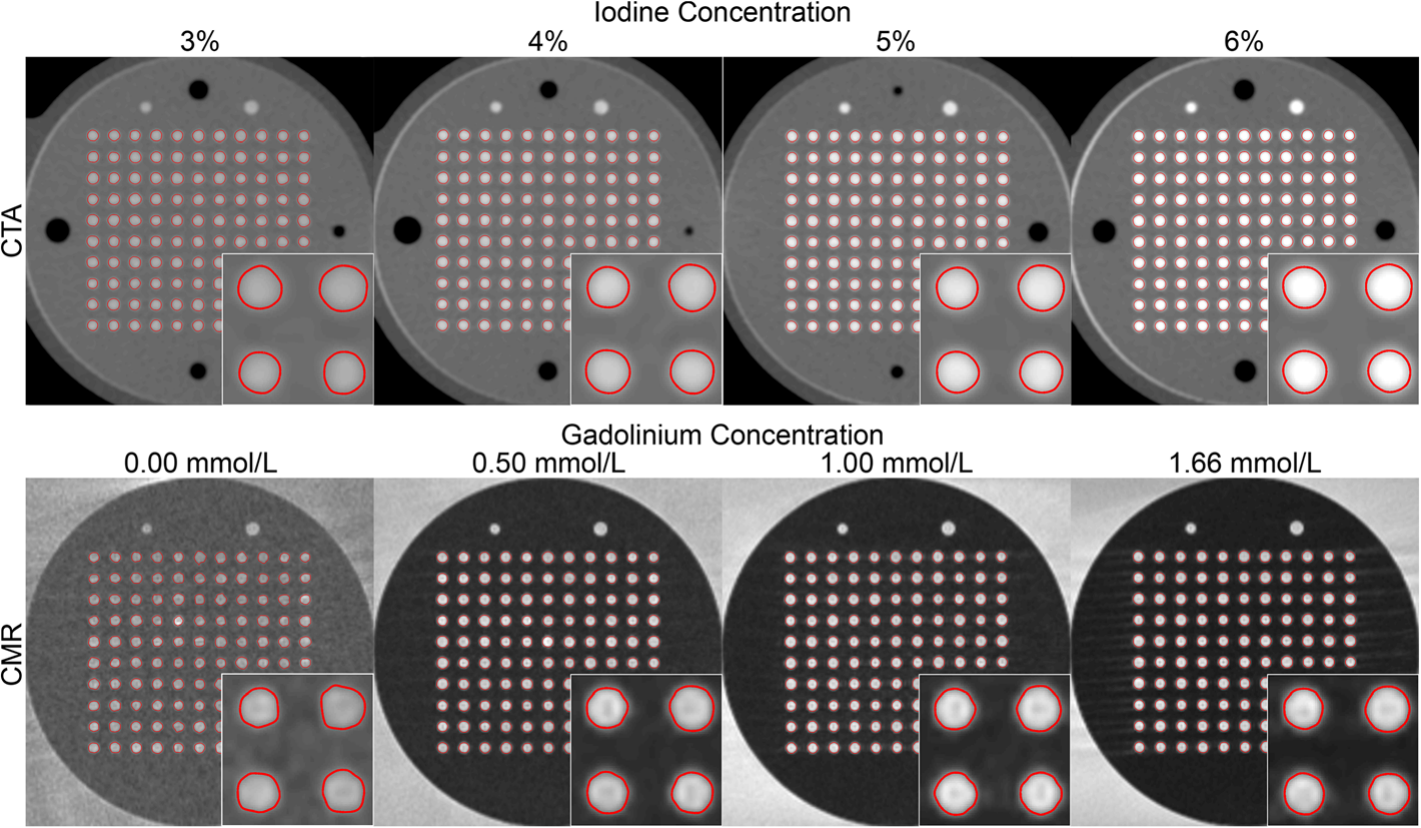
Comparison of representative segmented computed tomography angiography (CTA) and cardiovascular magnetic resonance (CMR) images obtained using the optimized CTA protocol, i.e. 20 mGy, 100 kVp, and 90% ASiR-V. For clarity, only four of the eight gadolinium concentrations are shown for MR images, i.e., 0.00, 0.50, 1.00, and 1.66 mmol/L
